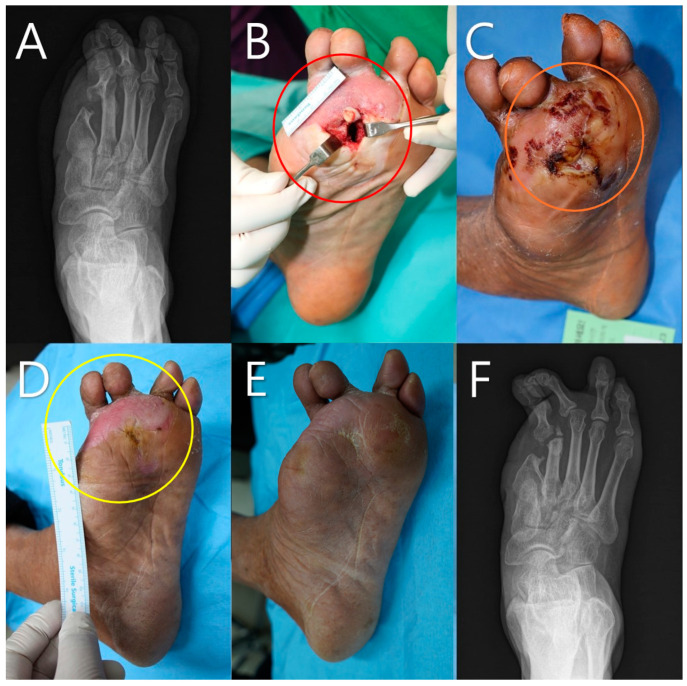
A 57-year-old male patient with a history of hypertension and diabetes. (A) Initial X-ray showing the extent of necrosis. (B) Intraoperative image showing escharectomy and necrotic tissue debridement, with red circle indicating the touching of bone and presence of *E. coli*. (C) Post-debridement image showing mild residual infection (orange circle) before micronized acellular dermal matrices (mADMs) application. (D) Wound after 20 days following a total of six mADM applications, showing significant healing with no signs of infection (yellow circle). (E) Wound at the 6-week outpatient follow-up, showing significant healing. (F) Final X-ray showing no signs of osteomyelitis.
